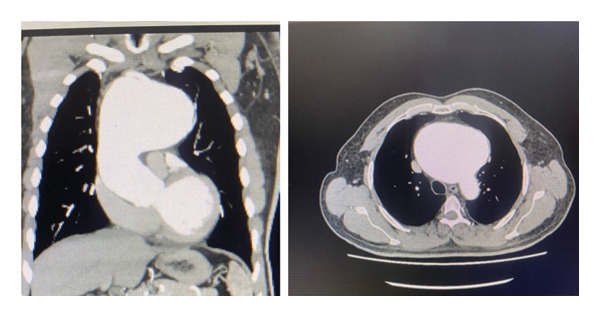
CT angiography images showing a large aneurysm involving the ascending aorta and the aortic arch.
